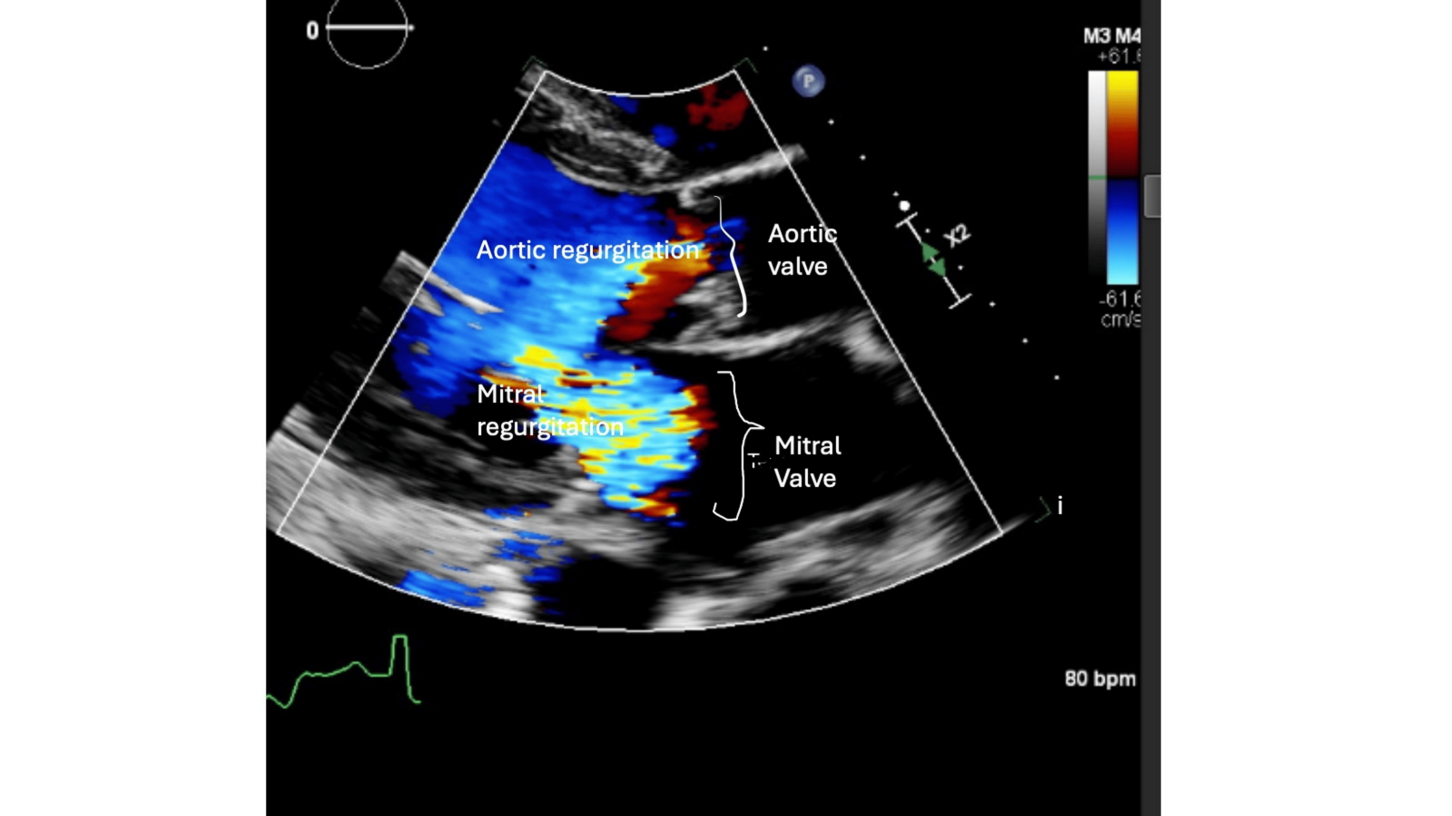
Transthoracic echocardiography image of aortic and mitral valve dysfunction and insufficiency (Doppler mode) These findings correlate with the patient's symptoms of dyspnea and orthopnea, indicating significant valvular disease.
